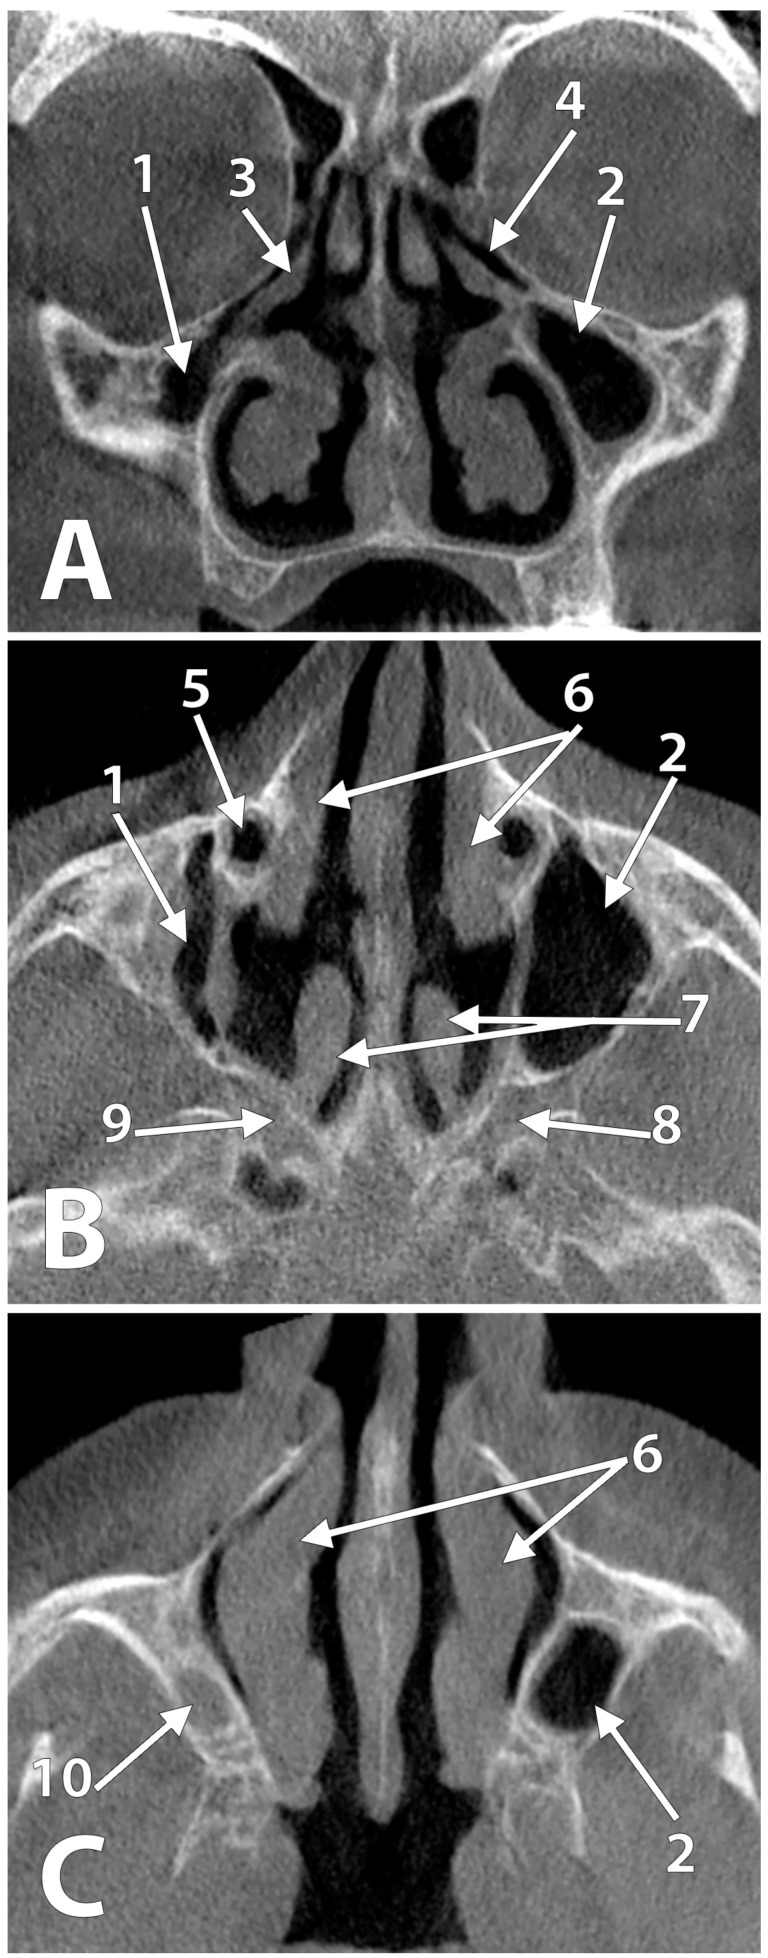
Planar CBCT slices through the hypoplastic maxillary sinuses. (A) Coronal slice through the second upper premolar regions; (B) axial slice through the roofs of the maxillary sinuses; (C) axial slice through the floor of the right maxillary sinus. (1) right maxillary sinus; (2) left maxillary sinus; (3) right uncinate process; (4) left ethmoidal infundibulum; (5) right aerated nasolacrimal duct; (6) inferior nasal turbinates; (7) middle nasal turbinates; (8) left pterygopalatine fossa; (9) right pterygopalatine fossa; (10) floor of the right maxillary sinus.
